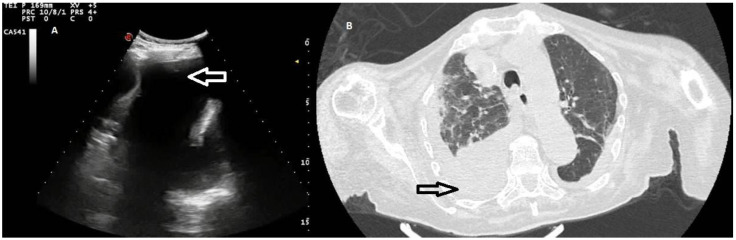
(A) TUS scan showing a complex nonseptated effusion with consensual parenchymal atelectasis. The tip of the needle during TUS-guided thoracentesis with a multifrequency convex probe (3.5 MHz) is highlighted by a white arrow. (B) The corresponding CT scan shows an extensive apico-parieto-basal pleural effusion of greater right expression (black arrow) with consensual lower lobe atelectasis. A bilateral thickening of interlobular septa and some right ground-glass opacities with partial sparing of the lung periphery are also present (congestive heart failure).
